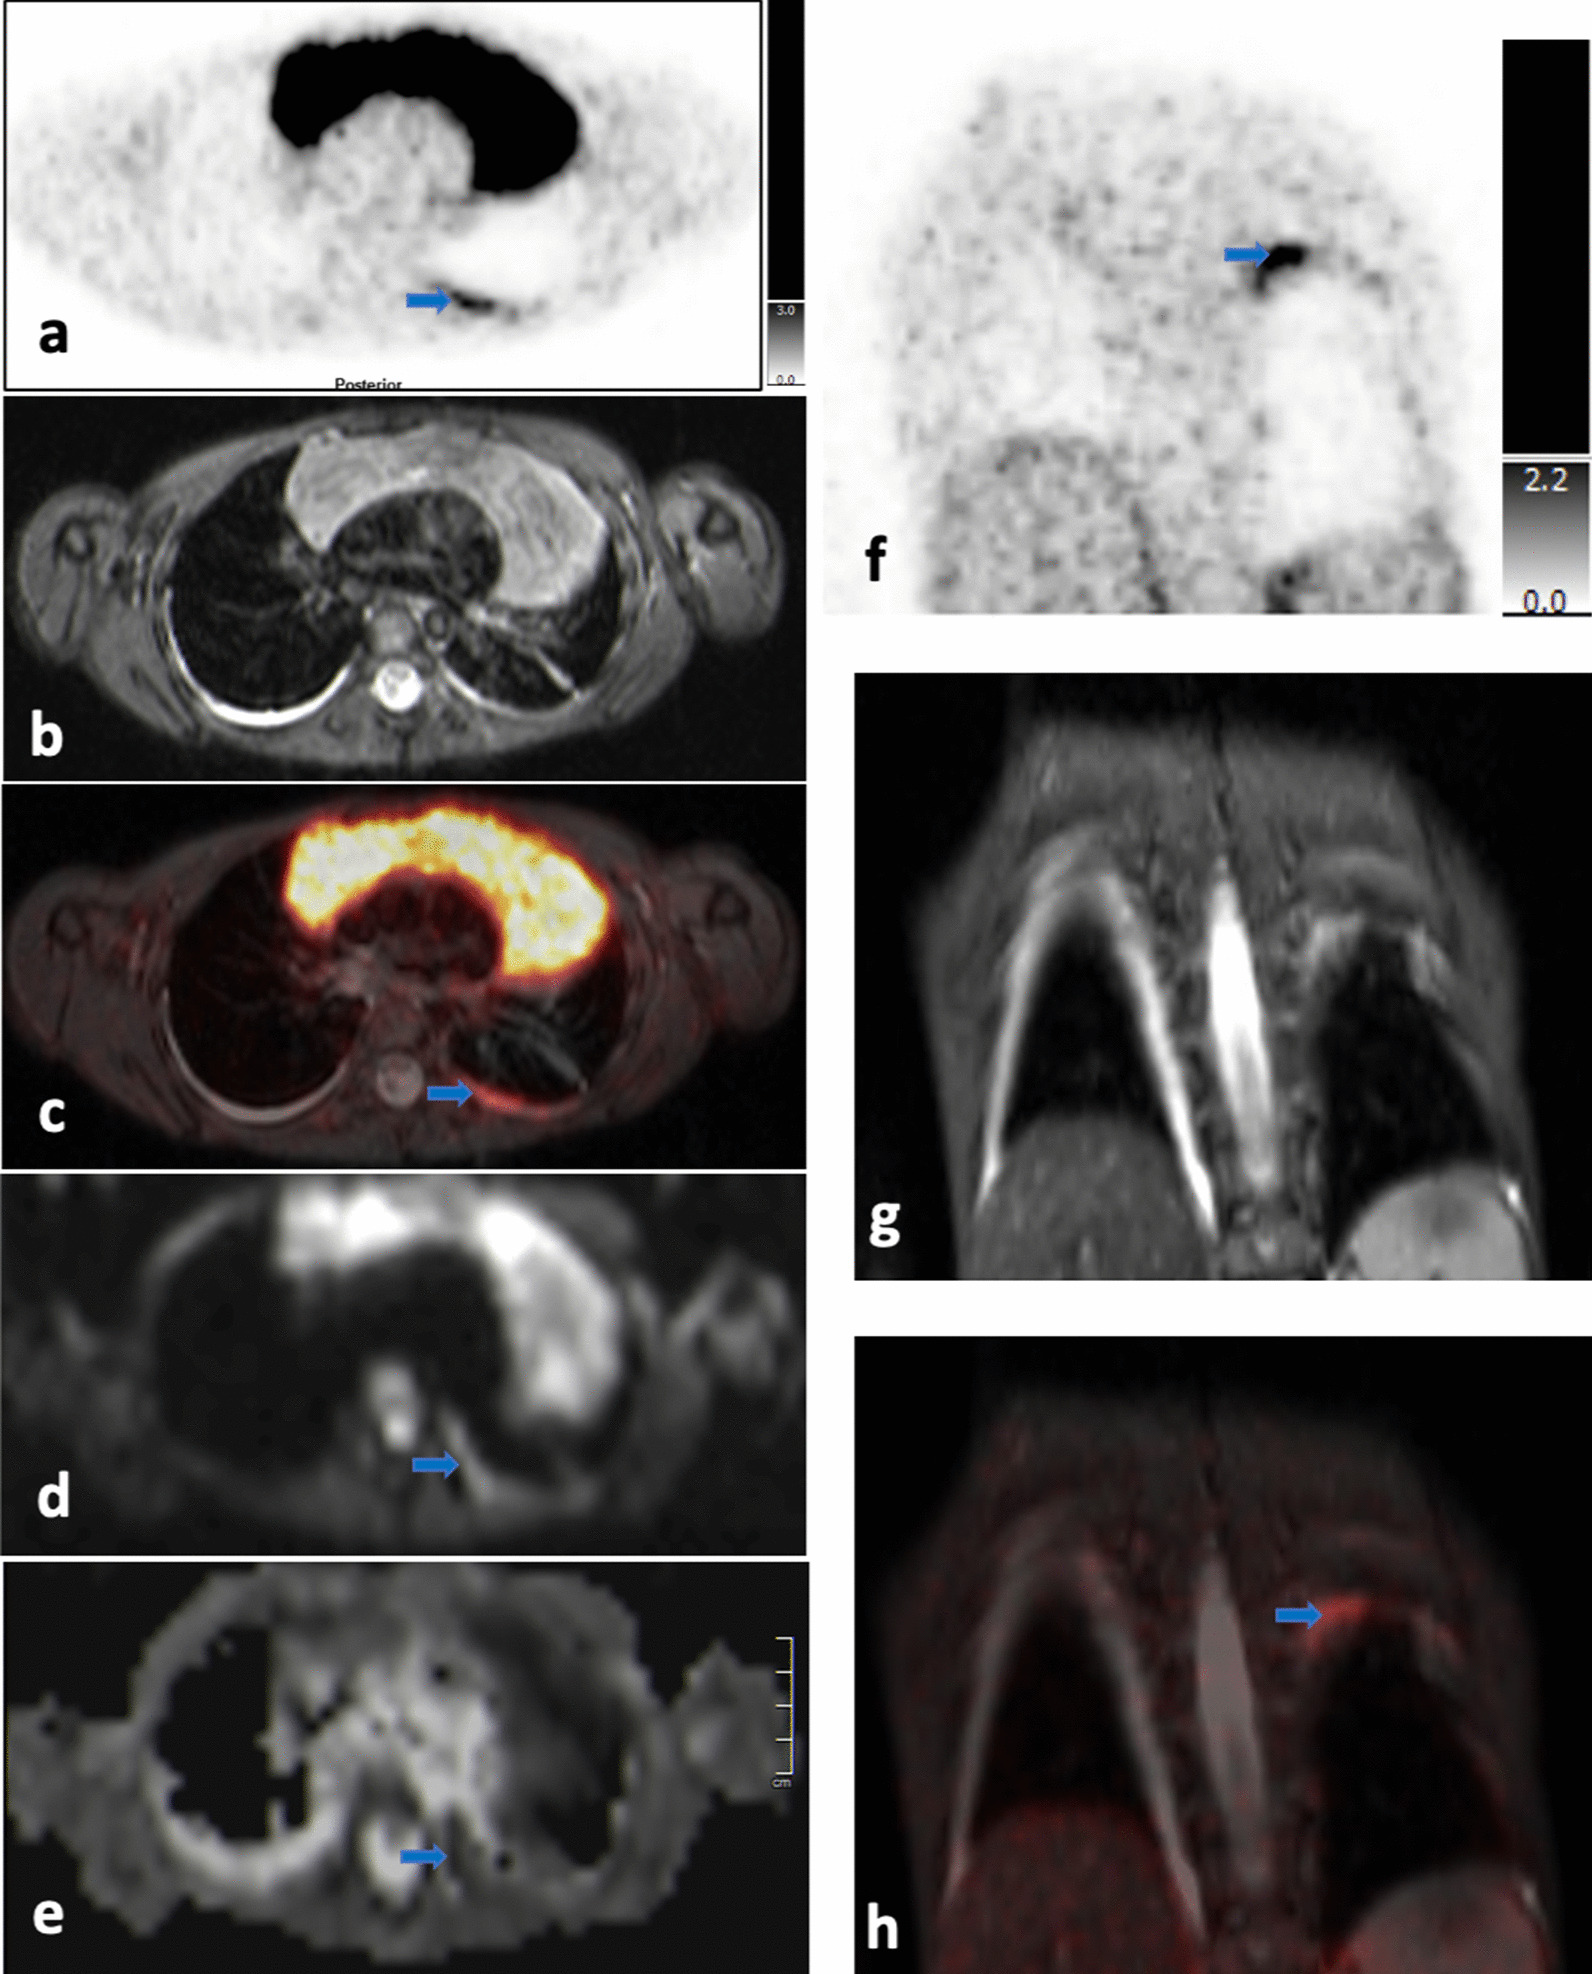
a–h [^18^F]-FDG-PET images transversal and coronal (a, f), MR T2-TIRM images transversal and coronal (b,g), [^18^F]-FDG-PET/MRI (c,h) and DWI at b-value of 800 (d,e) of the thorax. The blue arrows point to an area of the left posterior pleura with increased glucose uptake (SUV_max_ 2.88) (a, c, f, h) and a restriction in diffusion (ADC levels of about 636 × 10^–6^ mm^2^/s) (d, e). This area corresponds to pleura involvement which is not distinguishable from pleural effusion on MR T2-TIRM images (b, g)
